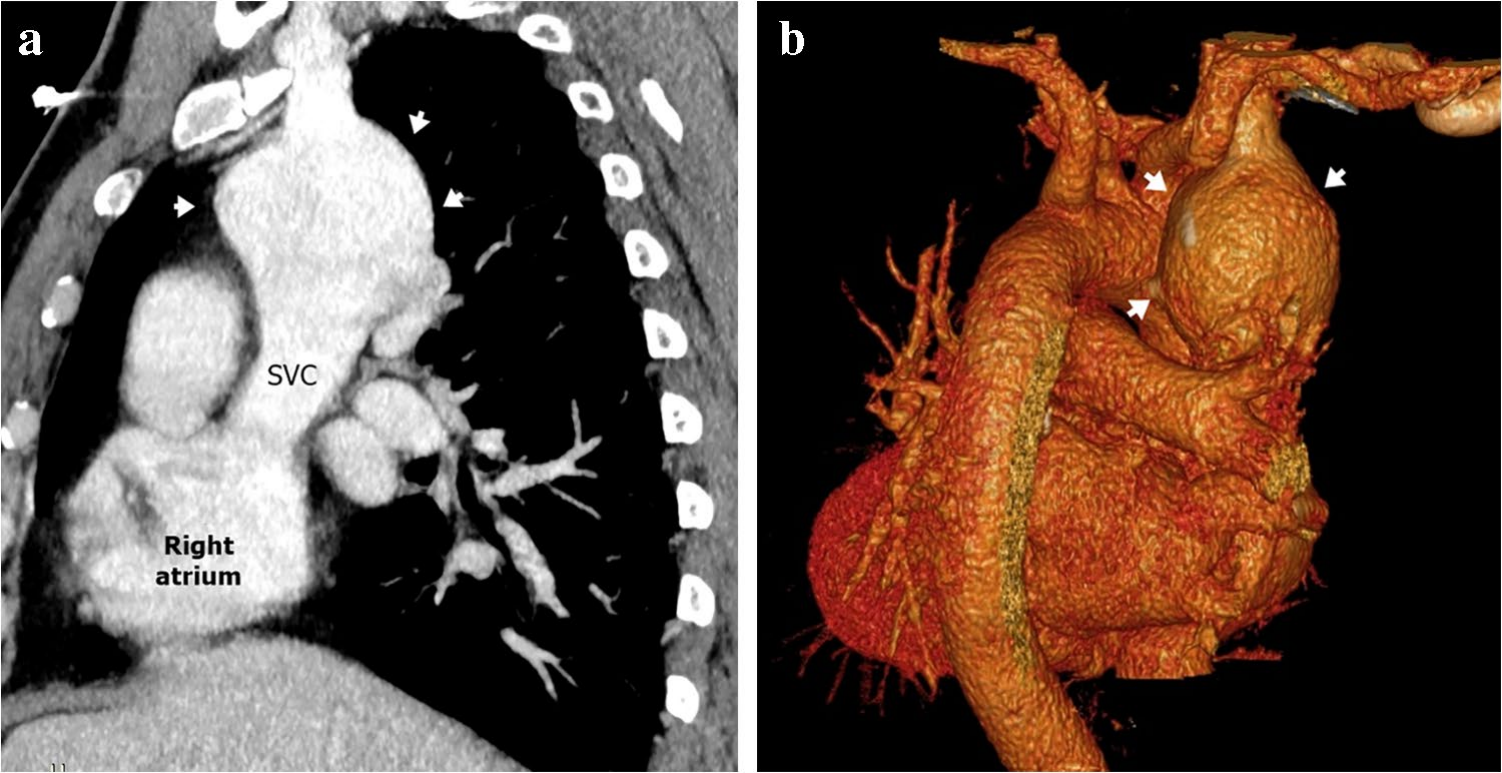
A right superior vena cava (SVC) aneurysm in a 62-year-old man. a, b Sagittal oblique computed tomography (CT) image (a) and volume-rendered CT image (b) show fusiform aneurysmal dilatation of the SVC (*arrows*)
